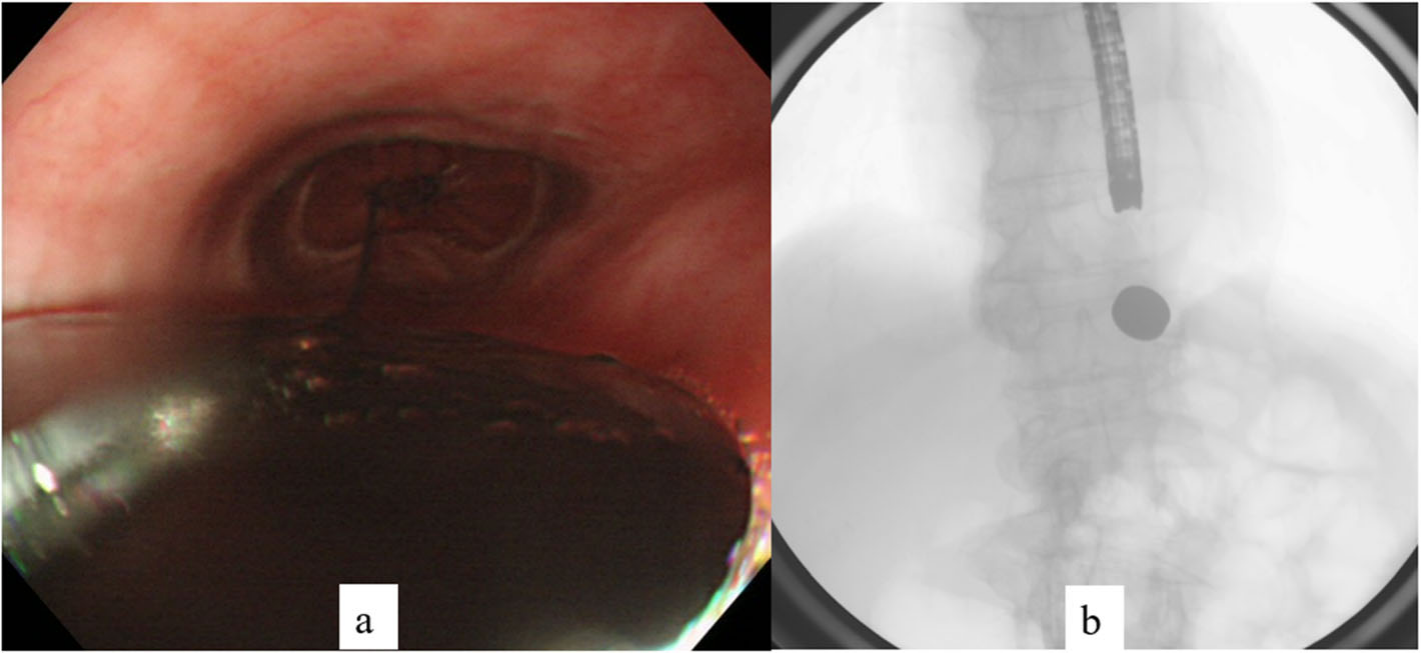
Magnetic compression anastomosis (placing the parent magnet on the anal side of the stenosis). a Esophagogastroduodenoscopy. b Radiograph
